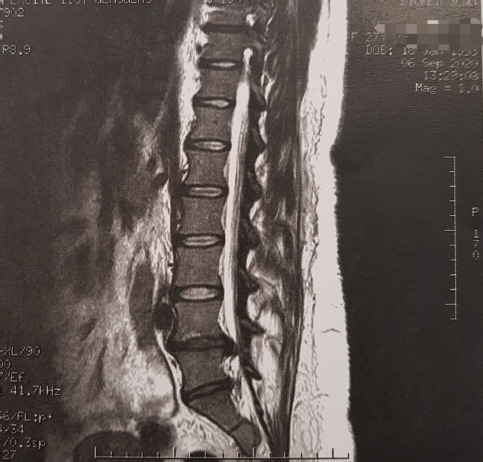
T2-weighted MRI scan, sagittal section, showing a large L4/L5 lumbar disc herniation with a caudally-migrated disc fragment.
